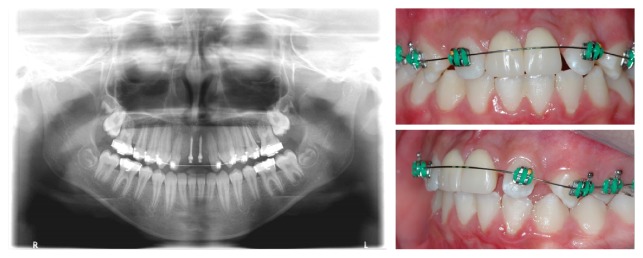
Clinico- and radiographic aspects one year after mini-implant placement.
